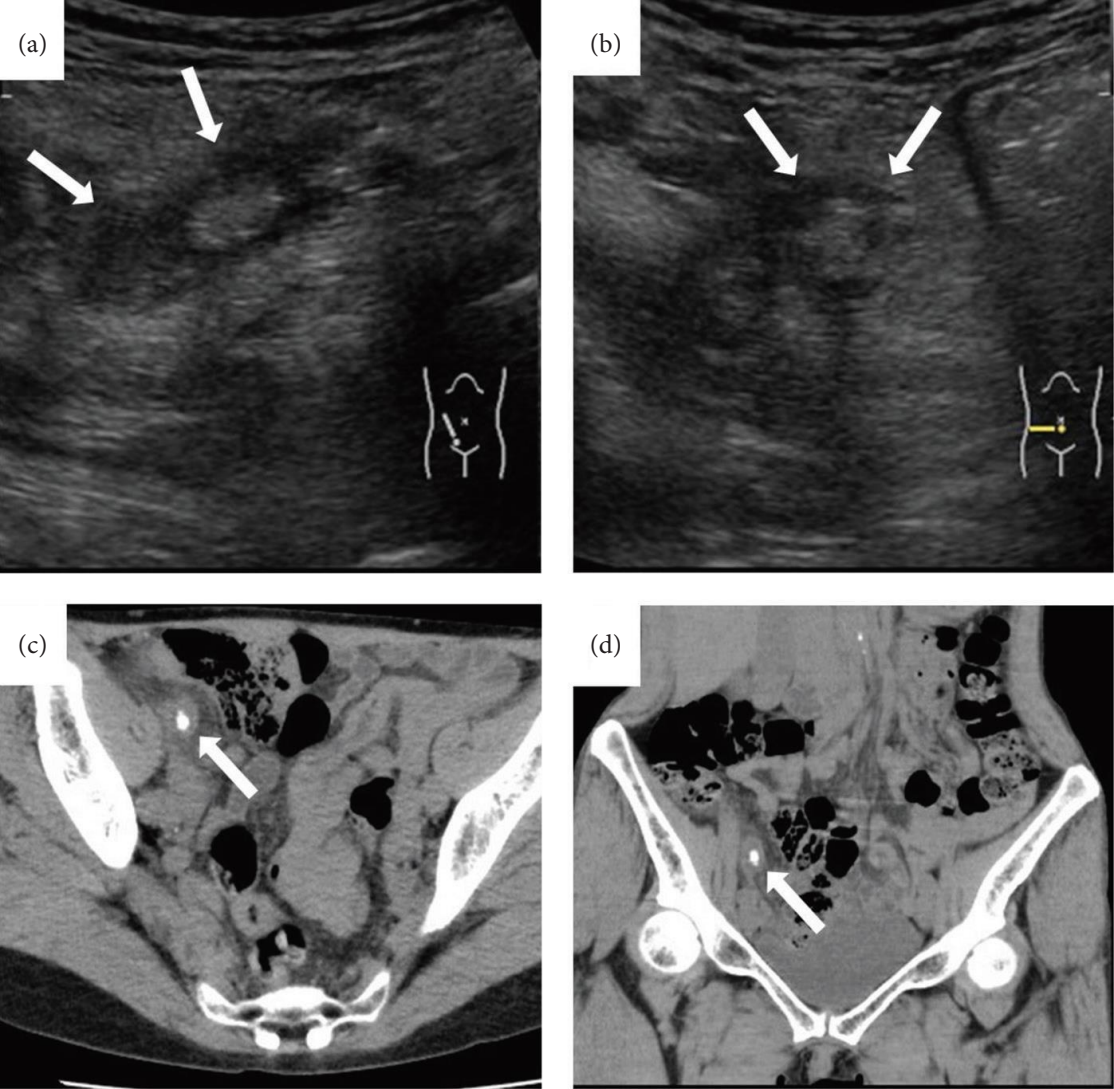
Abdominal ultrasonography and abdominal CT findings on preoperation. (a, b) Abdominal ultrasonography showed a swollen appendix and fecalith in the appendiceal lumen (arrows). (c, d) Abdominal CT also showed swelling of the appendix with fecalith and a small amount of fluid collection around the appendix (arrows) but no obvious free air.
